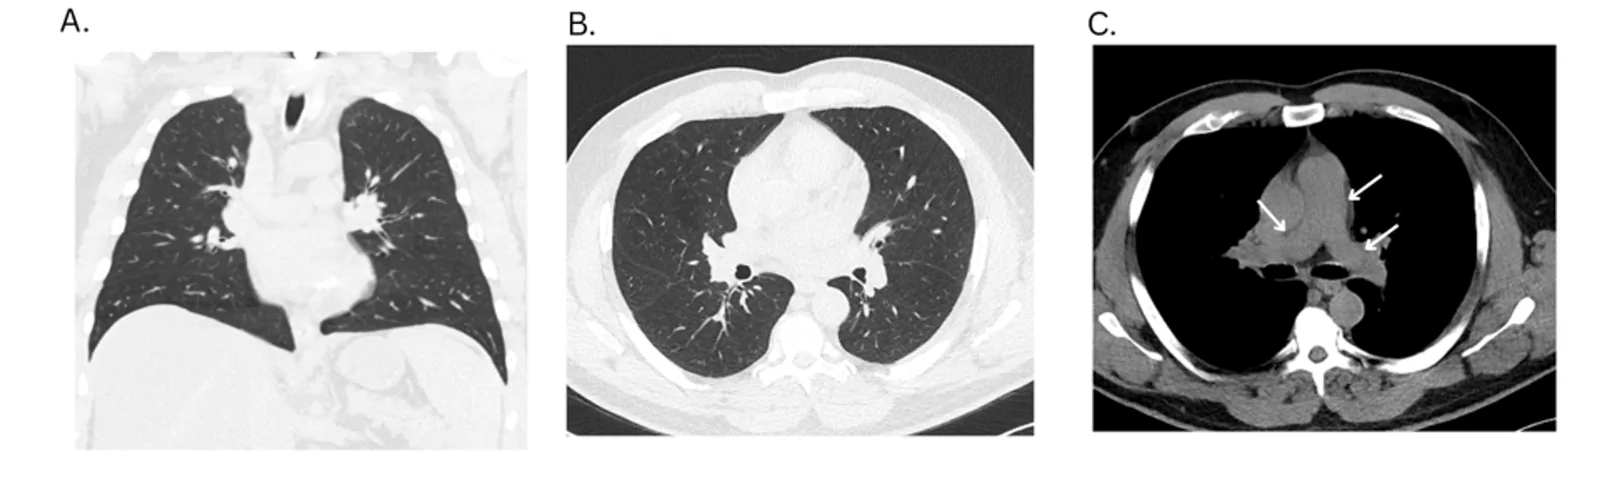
CT imaging findings in Case 2. Coronal and axial computed tomography (CT) images show enlarged central pulmonary arteries (C, arrows) and relative attenuation of the peripheral pulmonary arteries (A and B). Note the absence of mosaic lung attenuation.
